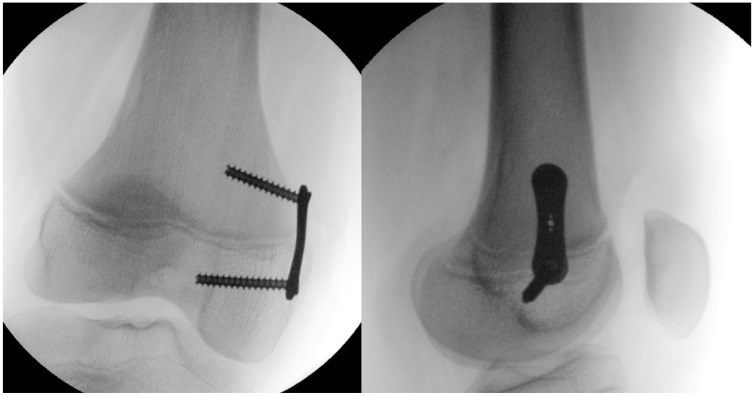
Postoperative biplanar radiographic evaluation of a temporary medial distal femoral hemiepiphysiodesis.
